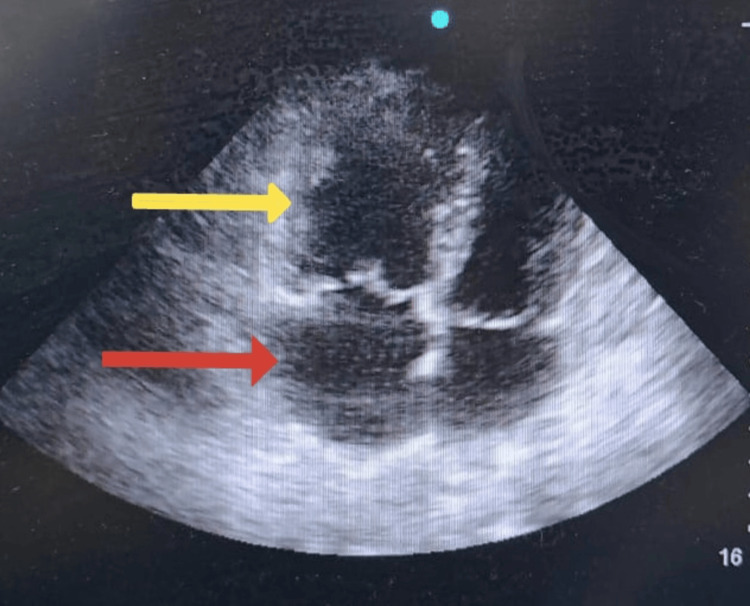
2D echocardiogram showing dilated right atrium (red arrow) and right ventricle (yellow arrow).
